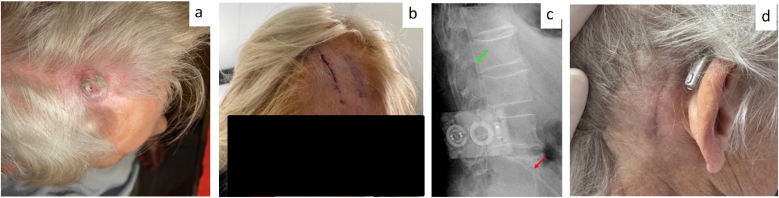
Representative Case 3: VPS-associated infection with radiodermatitis (single-staged LPS implantation) – secondary LPS implantation. 4a. CCWC after VPS-implantation with cranial exposure of the VPS after multiple revision surgeries. 4b. After superficial wound revision surgery with explantation of the VPS and implantation of an LPS. 4c. Postoperative X-ray following LPS implantation. The green arrow indicates the intrathecal spinal catheter; the red arrow indicates the peritoneal catheter. 4d. At 6-month follow-up, all wounds had healed sufficiently.
